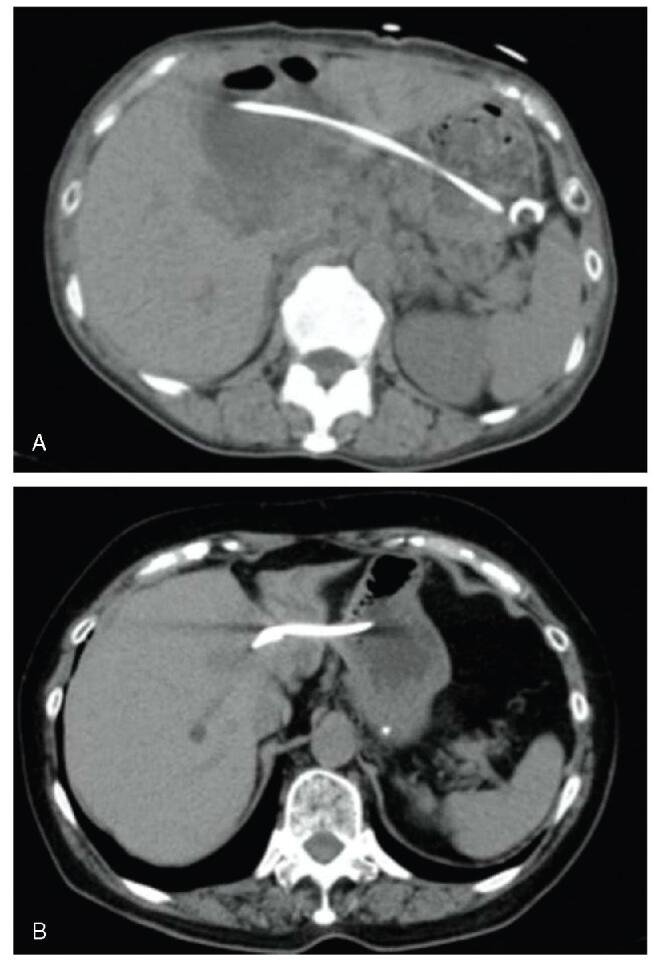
Figure 6. Abdominal plain computed tomography (CT) scans obtained after endoscopic ultrasound-guided drainage. A) CT imaging performed 4days after the procedure showing that the size of the liver cyst was decreased. B) CT imaging performed 3years after the procedure showing that the cyst has almost disappeared.
